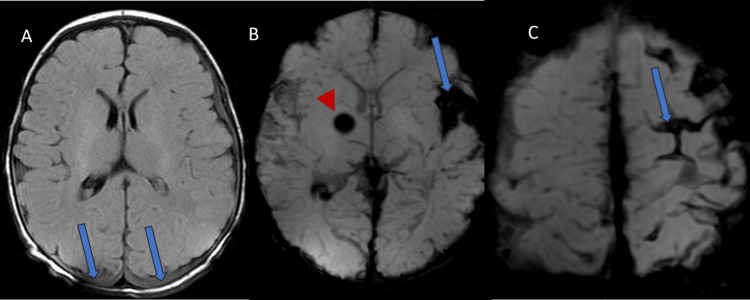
Unenhanced MRI of the brain: FLAIR axial image (A) shows bilateral subdural hemorrhages (arrows). Axial SWI, show right basal ganglia hemorrhage (arrowhead in B), subarachnoid hemorrhage (arrow in B), and subpial hemorrhage (arrow in C) FLAIR: fluid attenuation inversion recovery; SWI: susceptibility-weighted images
